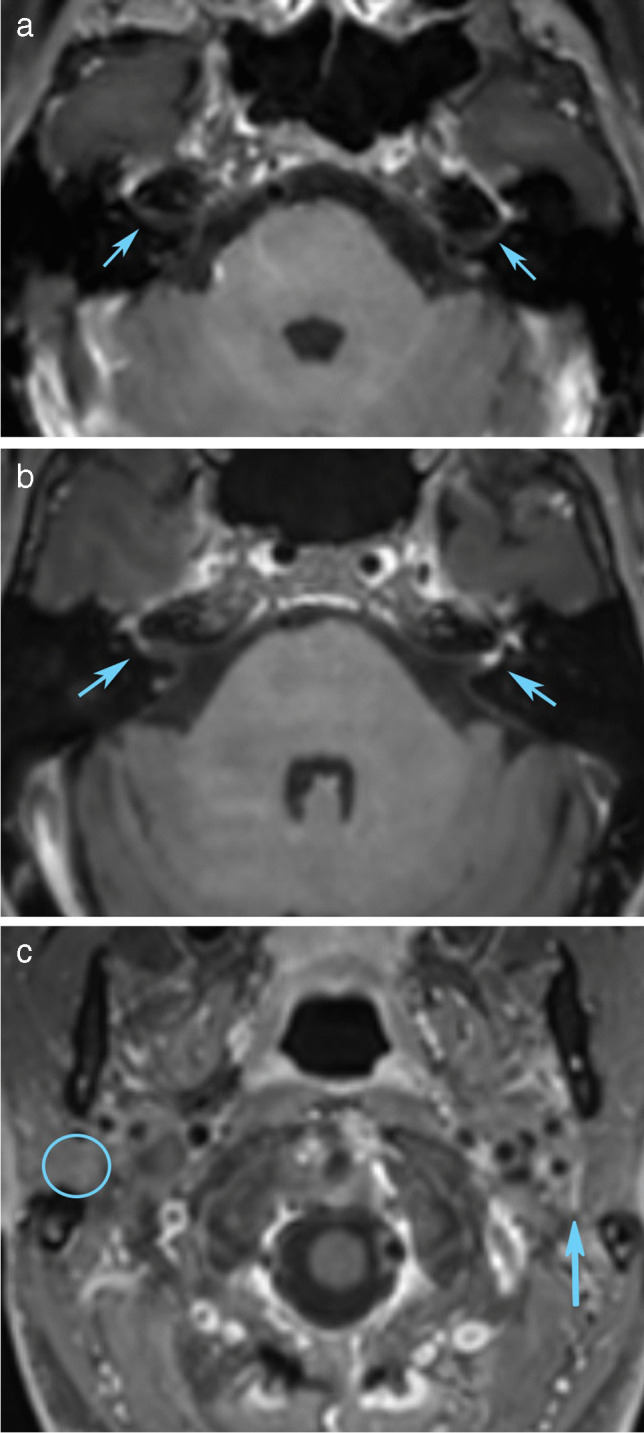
Evaluation of the facial nerve is complex due to a long course where some segments have physiological enhancement. Axial reconstruction from 3D T1-weighted and fat-suppressed acquisition shows no enhancement of the distal IAC segments (arrows in a), also there is no enhancement in the narrow labyrinthine segments that curve anterior toward the normal enhancing geniculate ganglions seen in the same axial slice. Axial images (b and c) are from a different subject at the level of the distal IAC and parotid gland, respectively. They demonstrate mild-moderate enhancement in the right distal IAC and strong enhancement in the left distal IAC (arrows in b), while no enhancement is seen in the right parotid segment (circle in c) and strong enhancement is present in the left parotid segment where the nerve is depicted as it curves anterior to approach the pes anserinus (arrow in c)
